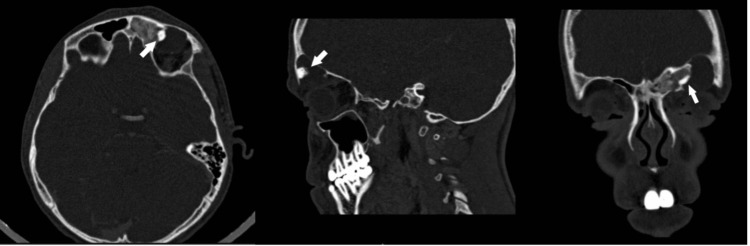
Preoperative CT scan Left frontal sinus osteoma (arrow) in axial, sagittal, and coronal views.
